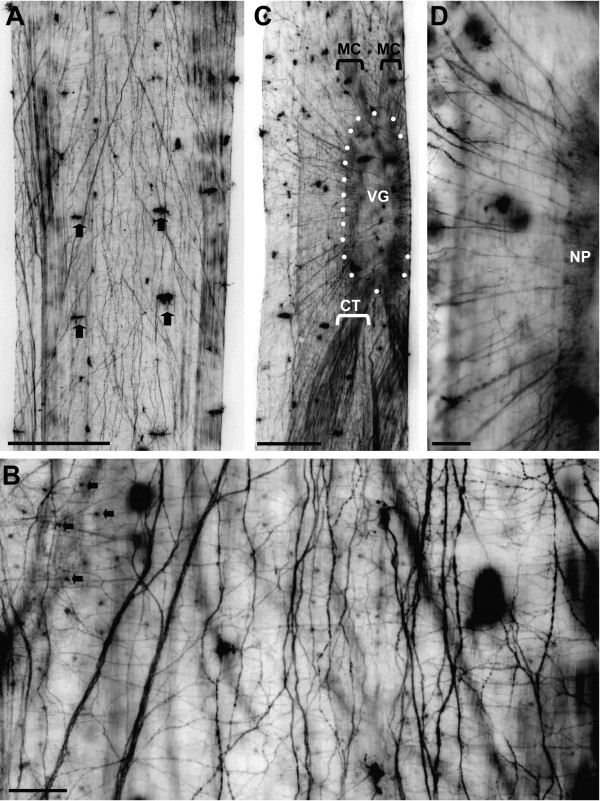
Whole mounts of adult *Sagitta setosa *labelled for acetylated alpha-tubulin (all imaged are black-white inverted). A: low-power dorsal view of the trunk surface (slightly posterior to the level of the ventral nerve centre) to show the intraepidermal nerve plexus. Arrows identify ciliary fence receptor organs. Scale bar: 250 μm B: higher magnification of the intraepidermal plexus. Arrows identify concentrations of labelled material that are the origin or target of very fine fibres and may correspond to peripheral multipolar neurons. Scale bar: 30 μm C, D: An irregular array of ca. 20 – 30 tubulin-labelled fibres and fibre bundles emerges laterally from both sides of the ventral nerve centre as a major source of the peripheral nerve plexus. Abbreviations: CT caudal tracts, MC main connective, NP neuropil, VG ventral nerve centre. Scale bars: 200 μm (C) and 60 μm.
